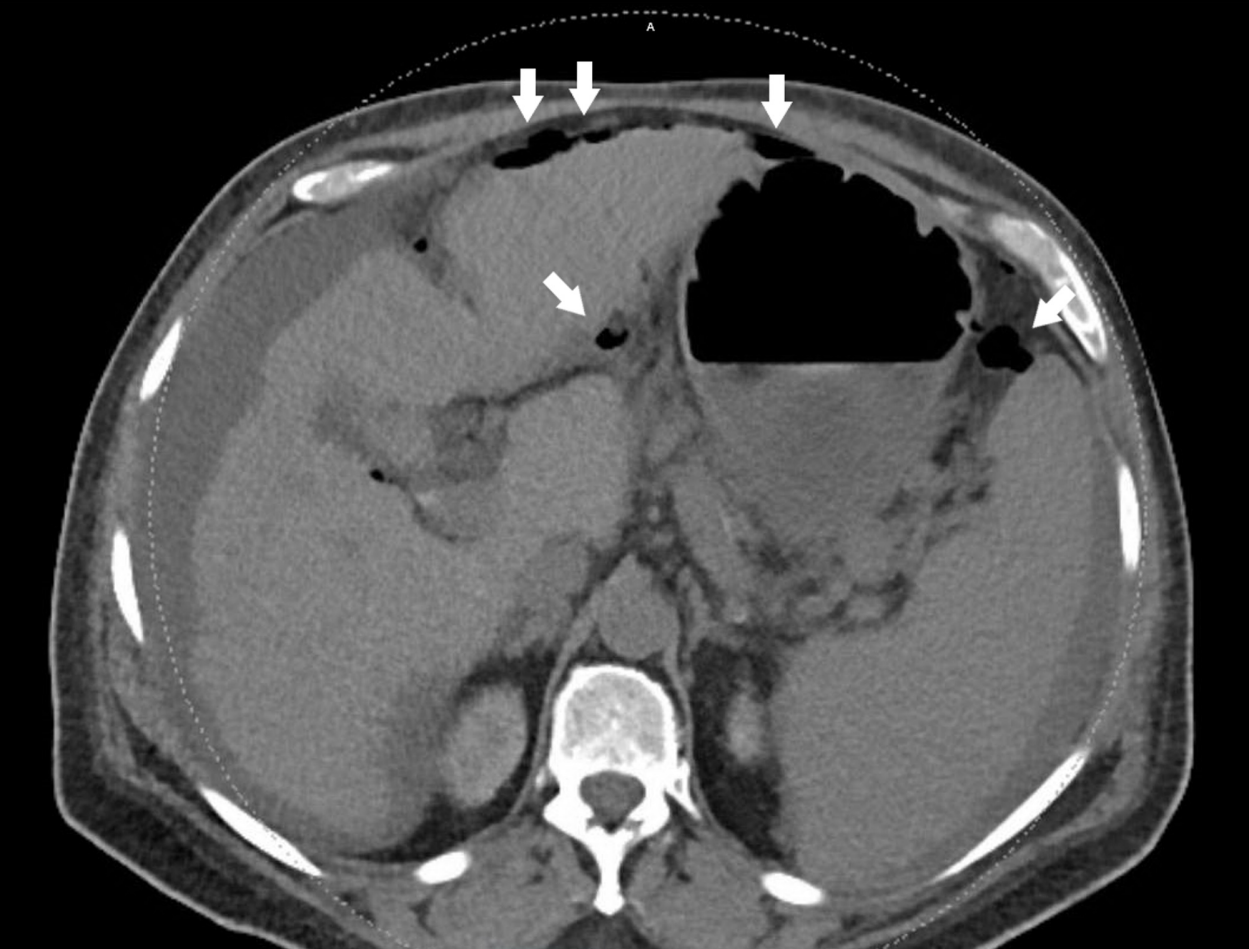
Axial noncontrast CT of abdomen demonstrating free air (arrows) around the stomach and anterior abdominal wall
